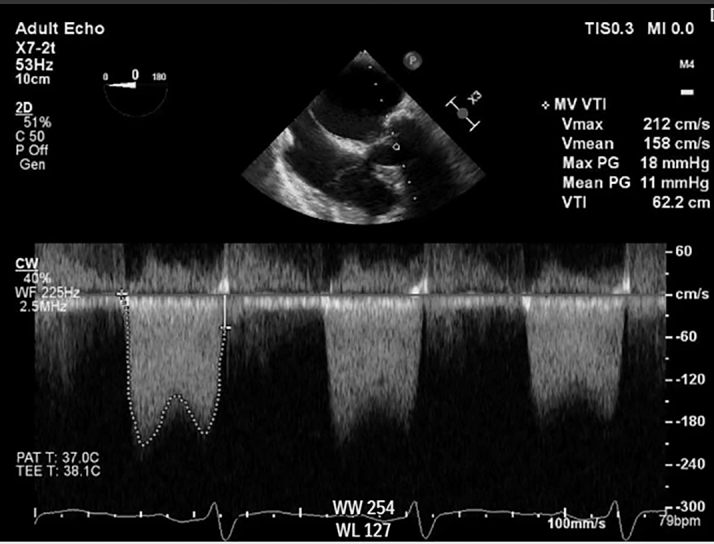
Transesophageal Echocardiography Showing Mean Mitral Valve Gradient 11 mm Hg at 79 beats/min
